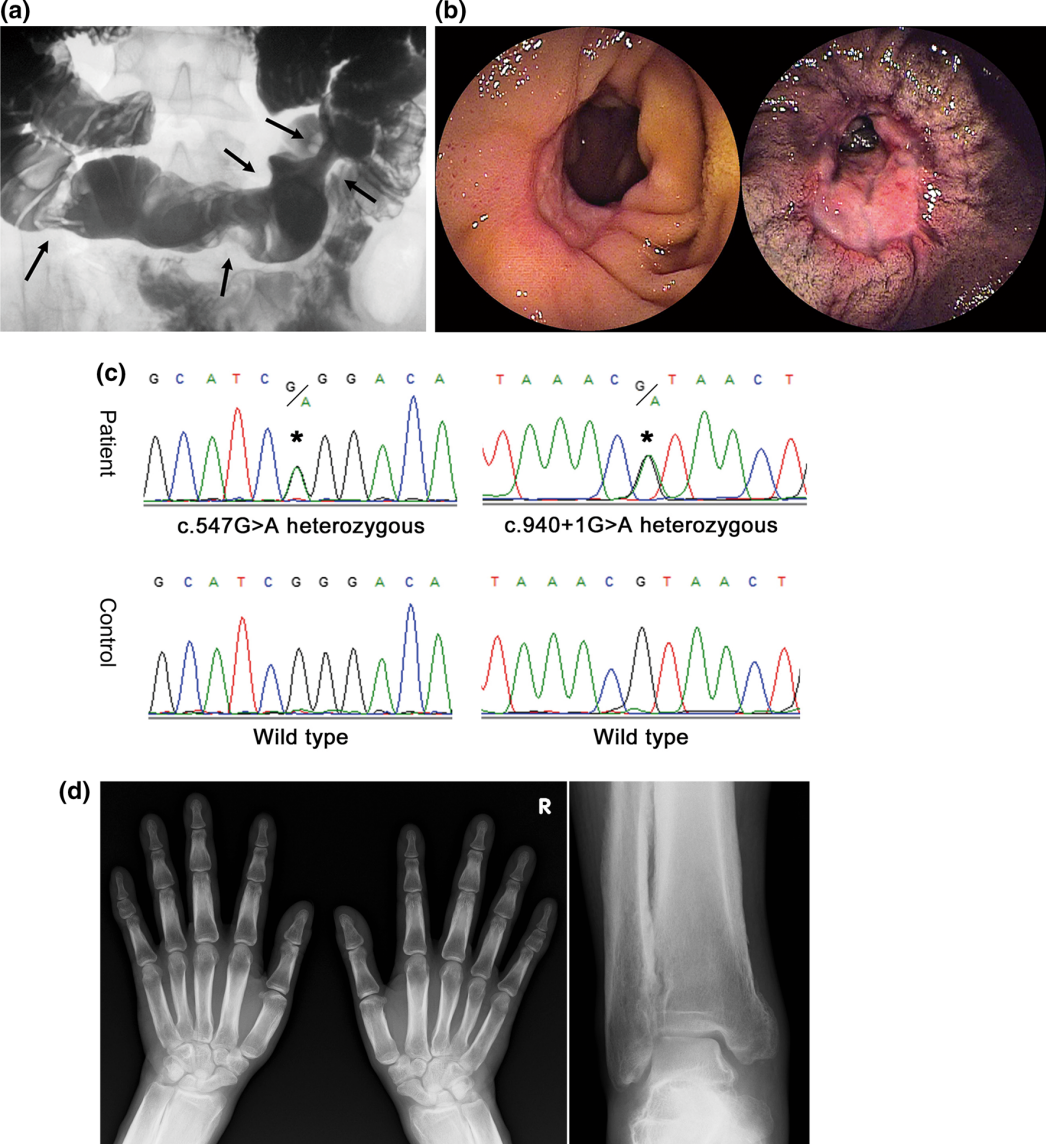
Clinical findings of a male patient (26 years) with CEAS and PHO. a Images of small bowel follow through. Filling image showing multiple eccentric rigidities in the ileum (*arrows*). b Images of double-balloon enteroscopy. Two lesions of ulceration with stenosis were observed in the ileum. c Sanger sequencing of the *SLCO2A1* gene. This patient had compound heterozygous mutations, namely c.547G > A (p.G183R) and c.940 + 1G > A (splice site). d X-ray images of the extremities. Radiographs of both the hands and ankle joints revealed cortical hyperostosis and periosteal reaction. Figures are reprinted with permission from Ref. [17] and [18]
